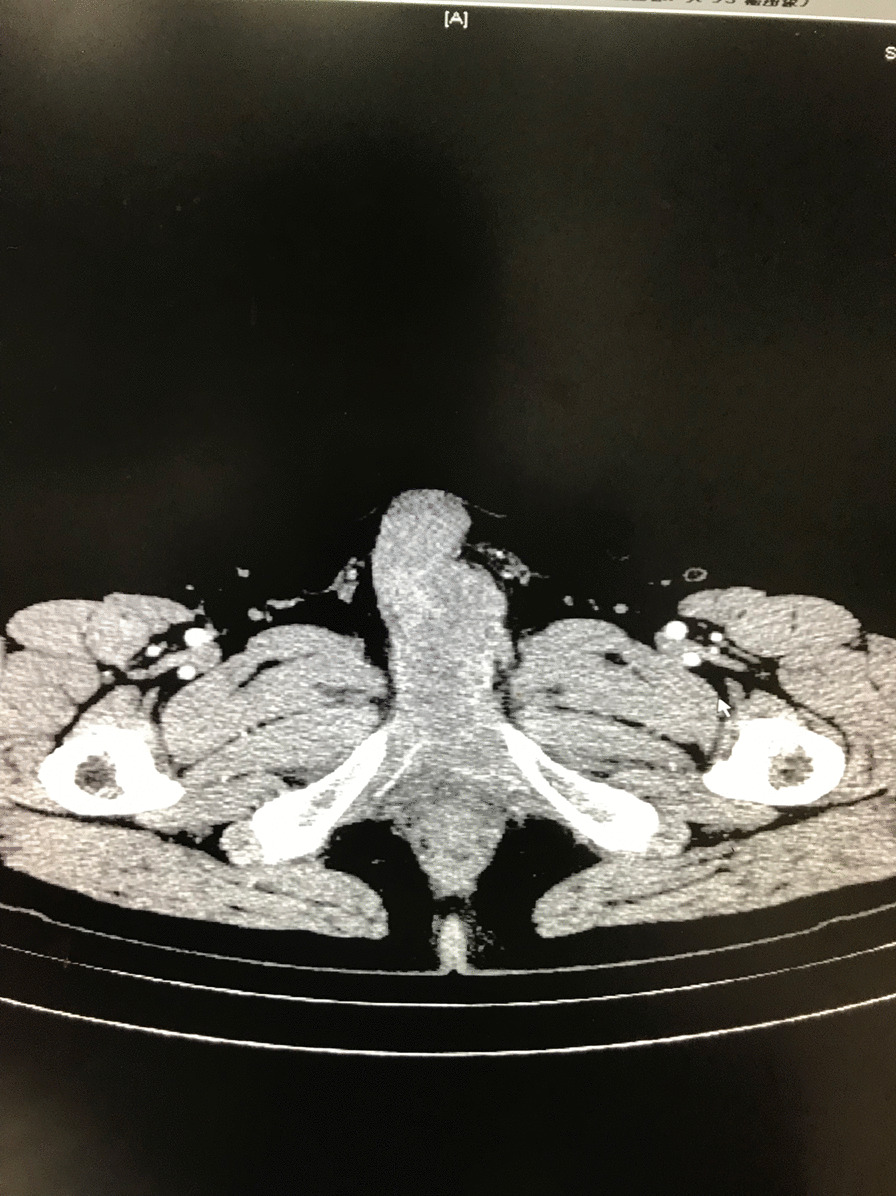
Computed tomography imaging. 3 months after surgery
